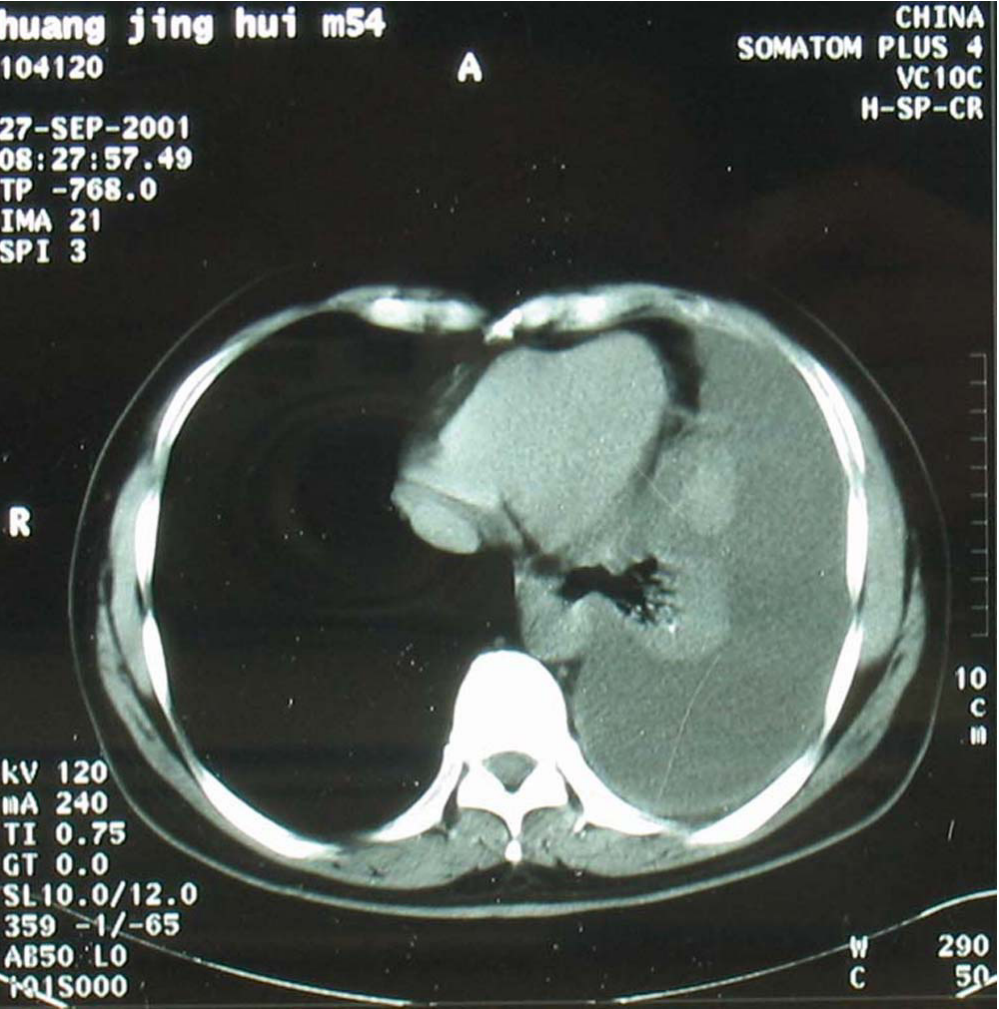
Chest CT before treatment (27-Sep-2001) show that conglomeration of a size of 5.5 × 4.2 cm at the left lower hilus pulmonis, large amount of accumulation of fluid in the left thoracic cavity, enlarged lymph nodes in the mediastinum.
